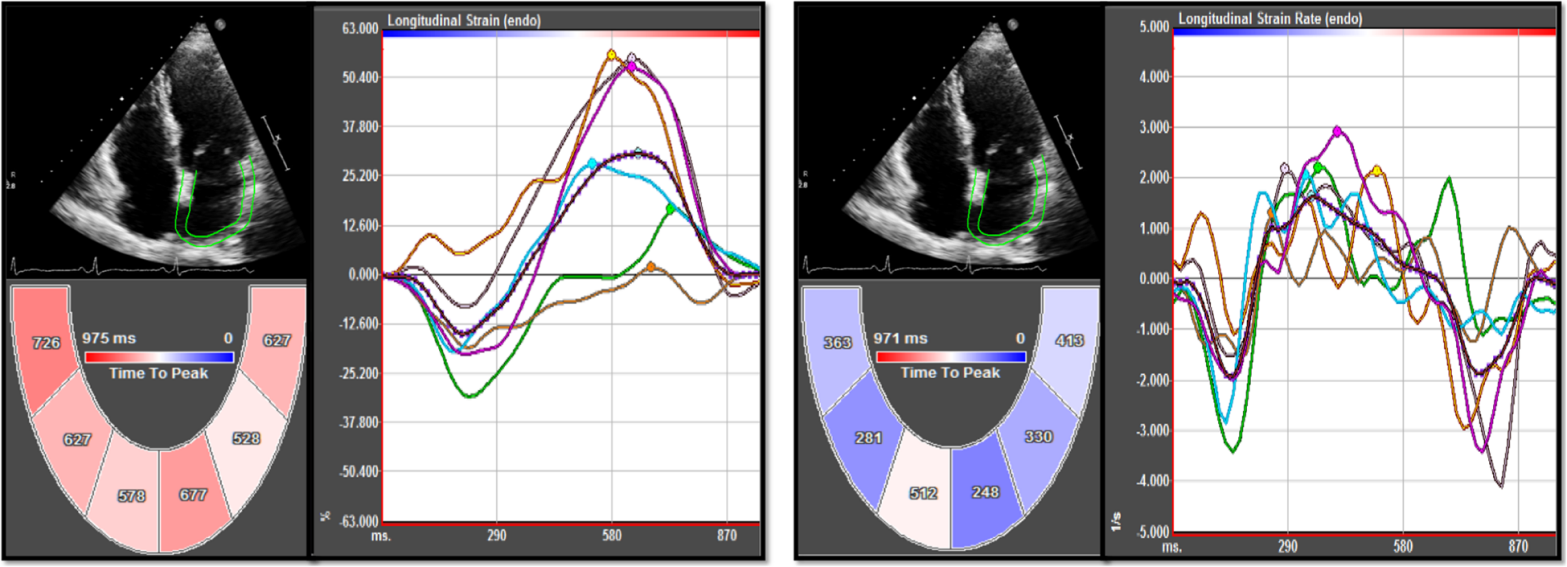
Pattern of left atrial strain (left) and strain rate (right) curves obtained from the apical four-chamber view in an example subject of our study population
